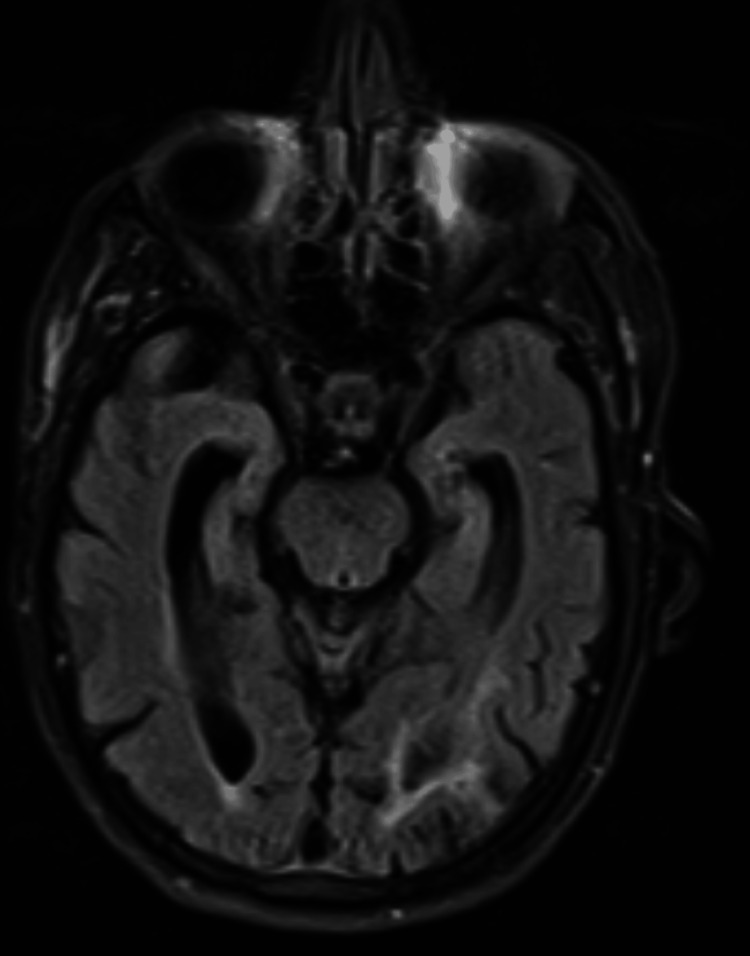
Negative fluid-attenuated inversion recovery (FLAIR) sequence of brain MRI at the level of the hippocampus.
